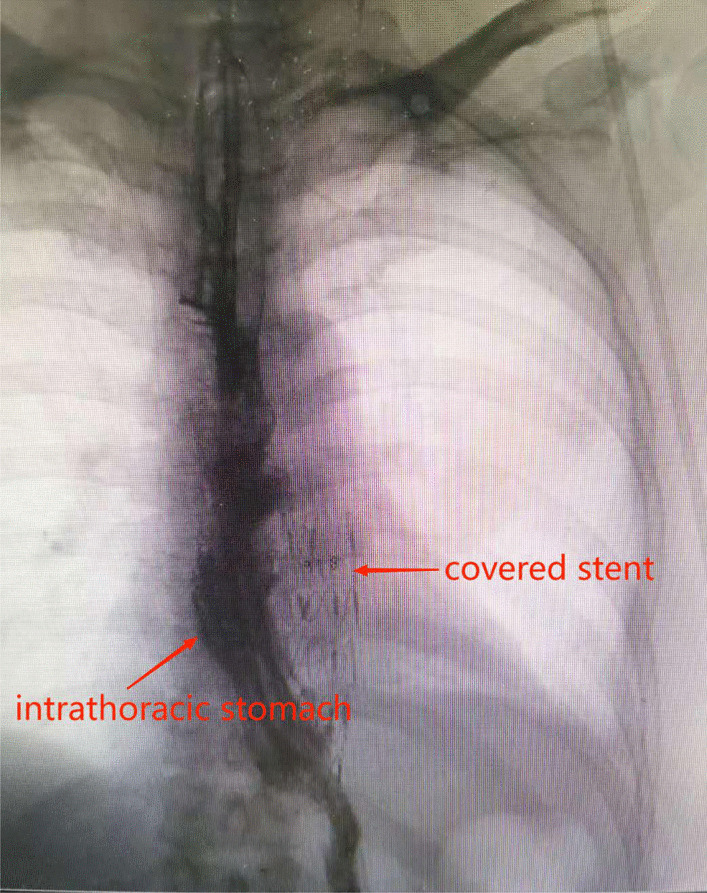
One-week after exploratory thoracotomy and TEVAR (Upper gastrointestinal radiography)
